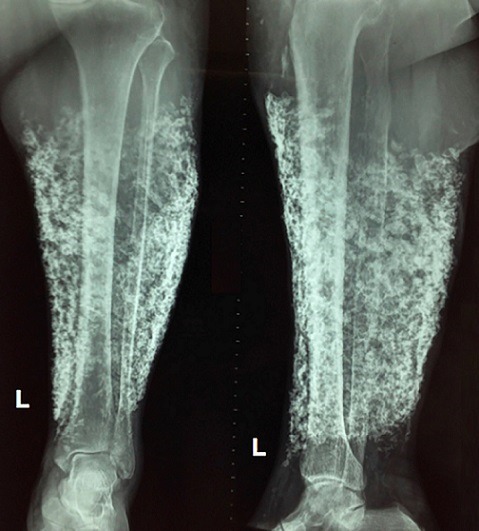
Radiograph of the left leg, demonstrating severe subcutaneous dystrophic calcification
